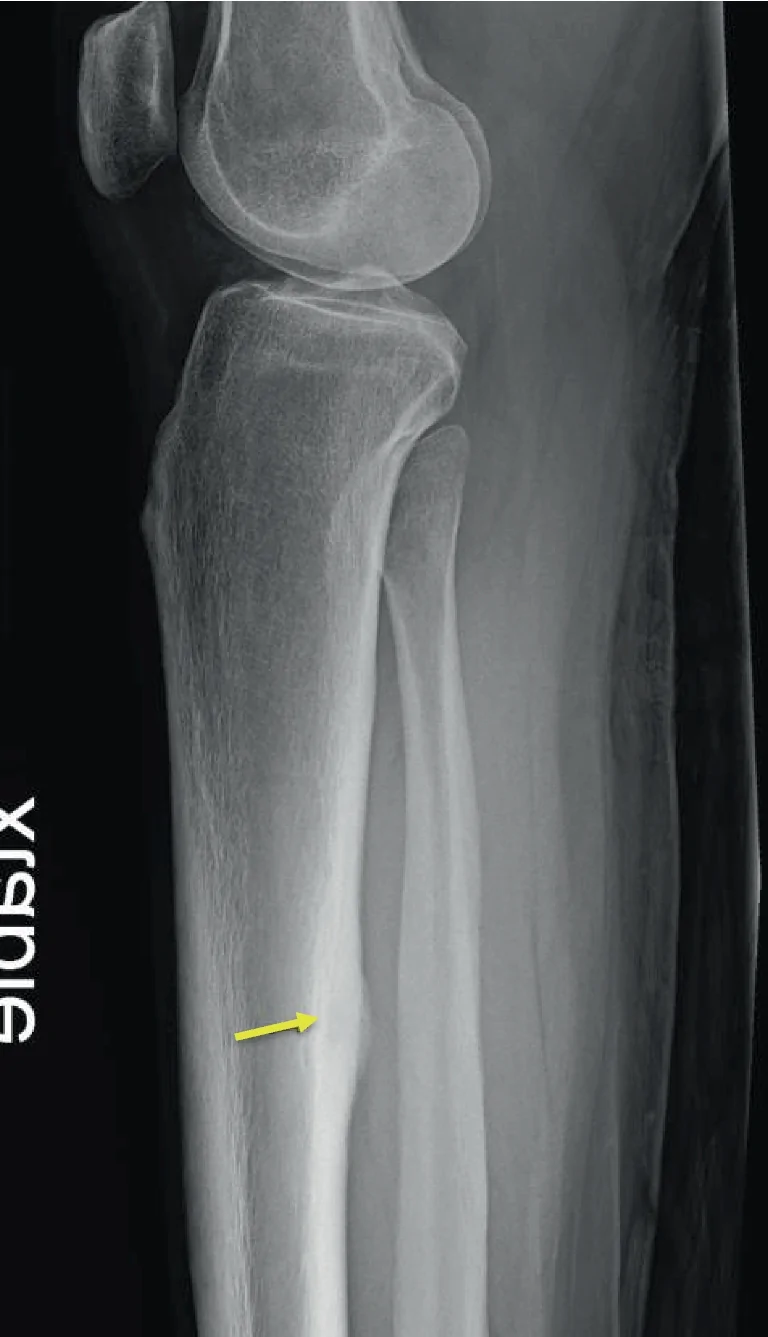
Lateral radiograph of the tibia and fibula with focal cortical thickening and periosteal bone formation of the posterior right tibial proximal diaphysis
